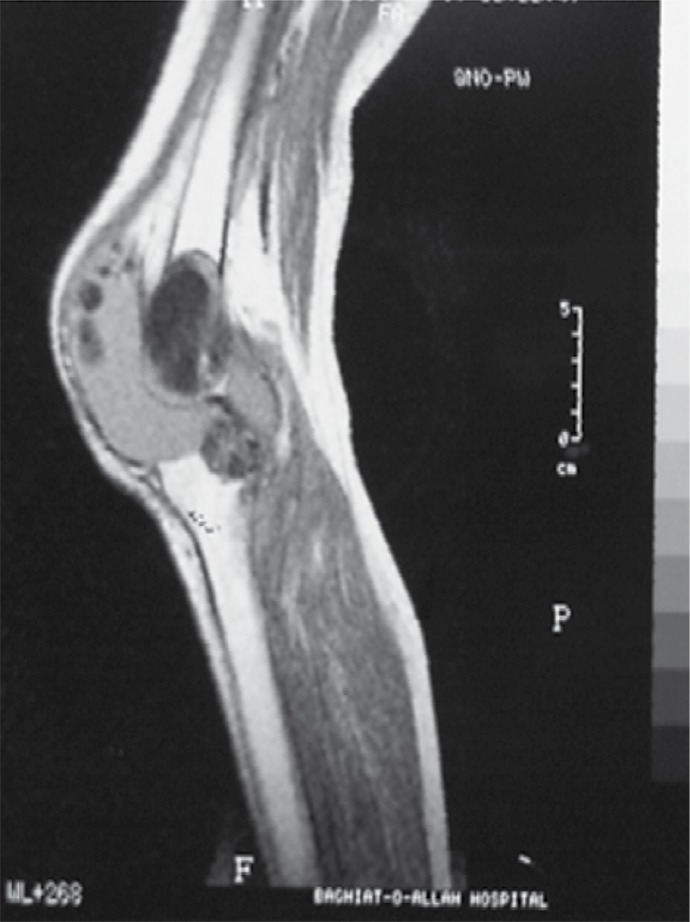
MRI (T1W-MRI) Without Contrast. It shows low signal intensity lesions in the proximal of the tibia and distal of the femur with joint space involvement.
